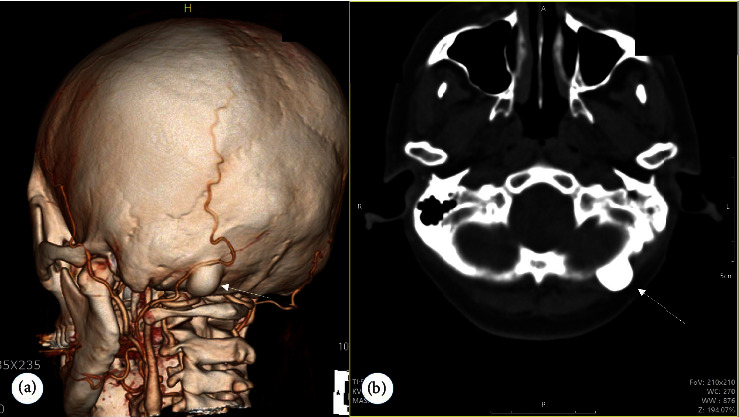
Computed tomographic (CT) scan images showing a single incidental suboccipital osteoma (arrow). A three-dimensional CT scan (a) and an axial CT image (b) of the osteoma. No association was found between osteoma and the greater occipital nerve.
